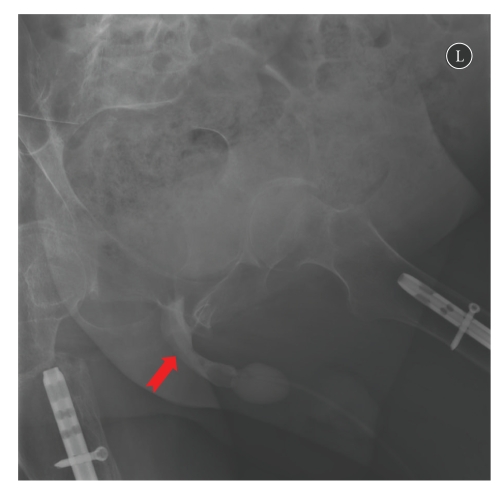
X-ray of pelvis: twenty mL of contrast was injected thorough lumen of Foley catheter. Proximal urethra was visualised by the injected contrast (arrow) thus confirming that the tip of Foley catheter was lying in urethra and not in the bladder. There was large amount of urine retained in the bladder.
